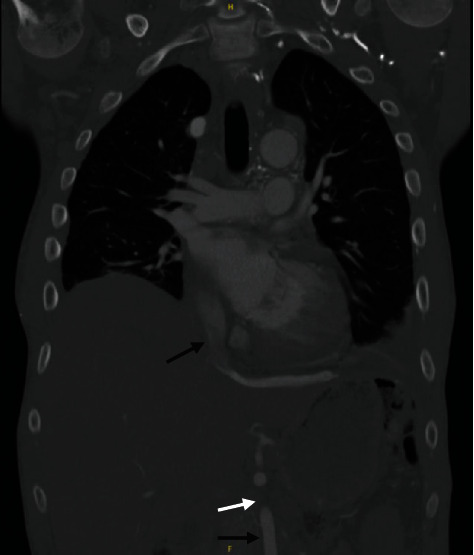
CT chest/abdomen/pelvis. CT scan demonstrating stenosis of a collateral to the IVC (white arrow). The IVC is indicated by the top black arrow. A collateral to the IVC is indicated by the bottom black arrow.
